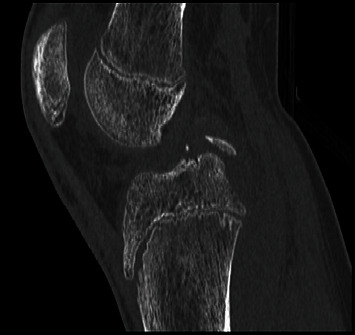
CT scan of case 1. The sagittal scan shows the displaced avulsed fragment.
